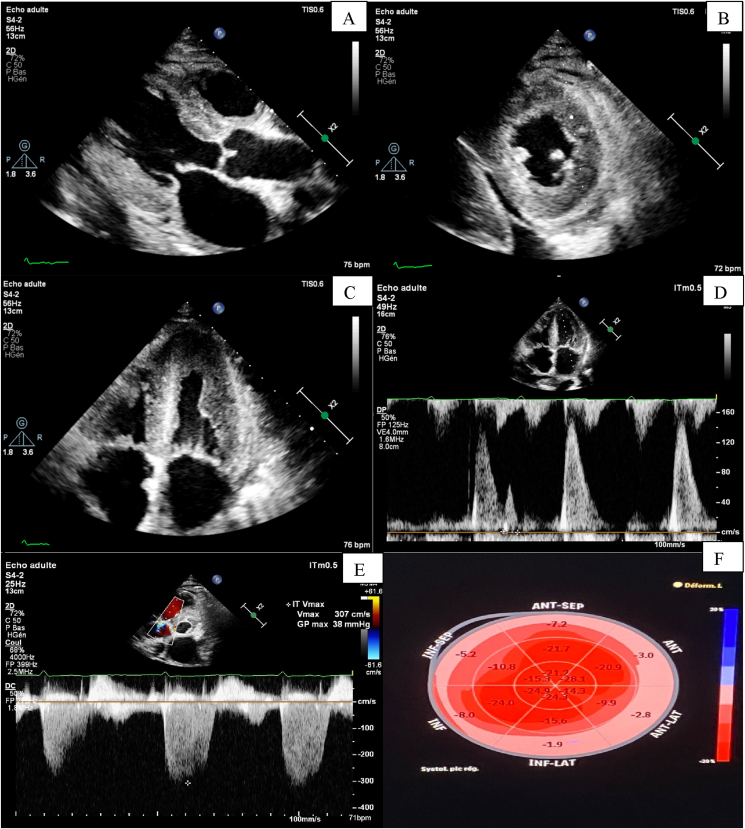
TEE findings: (A) parasternal long axis view showing shiny aspect of the interventricular septum with concentric wall thickening and a mild pericardial effusion. (B) parasternal short axis view showing an important concentric wall thickening of the left ventricle (C) Apical long axis view showing a biatrial enlargement associated with biventricular hypertrophy (D) Pulsed wave doppler of mitral inflow suggesting a restrictive mitral inflow pattern. (E) 2D-Strain showing the “bulls eye’’ appearance (F).
